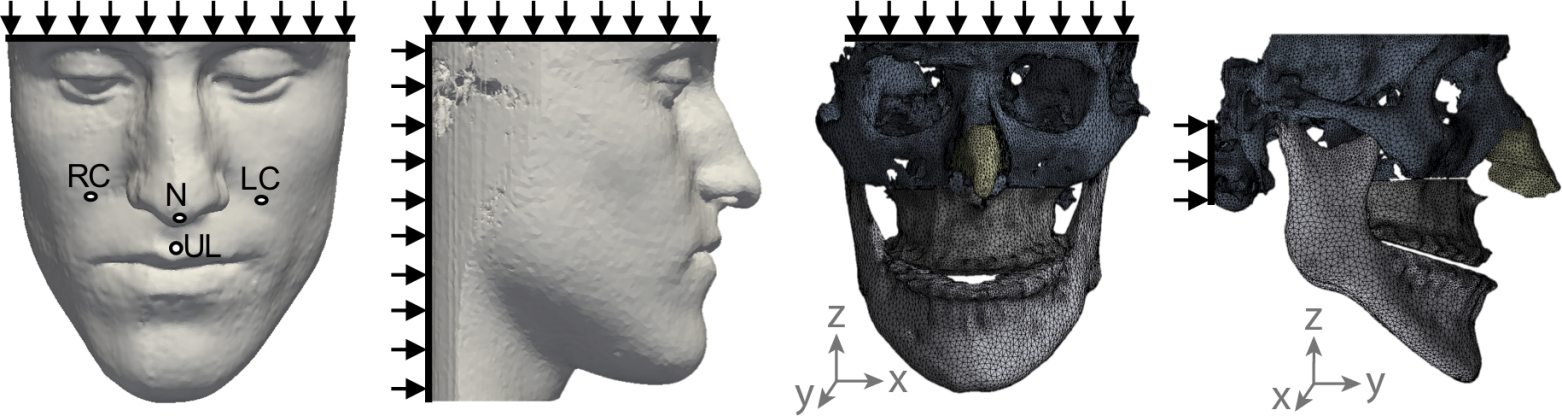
Preoperative CBCT reconstruction of the soft tissues and bones as well as boundary conditions. Boundary conditions are indicated by black arrows and the reference axis by the grey arrows. The soft tissue nodes evaluated in the design of experiments are also indicated: nose (N), upper lip (UL), lower lip (LL), right cheek (RC), and left cheek (LC).
